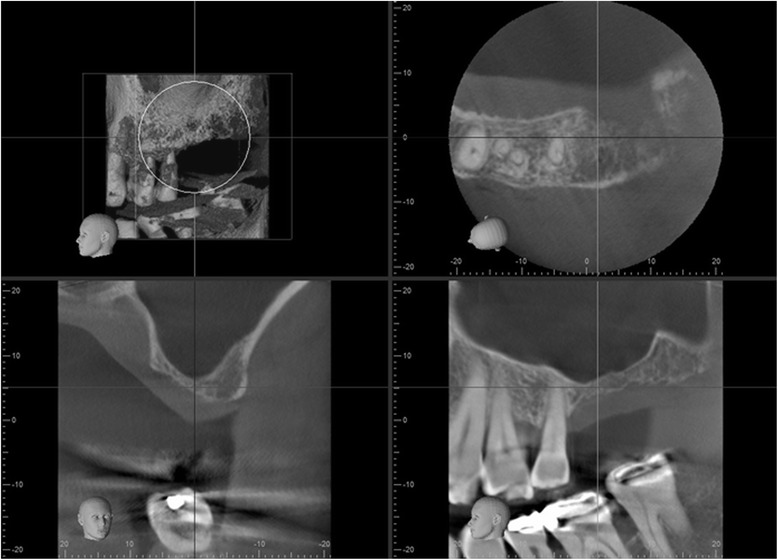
Left sinus prior to first sinus grafting procedure. Cone beam CT imaging also shows very little bone volume on left side for the no. 14 area
